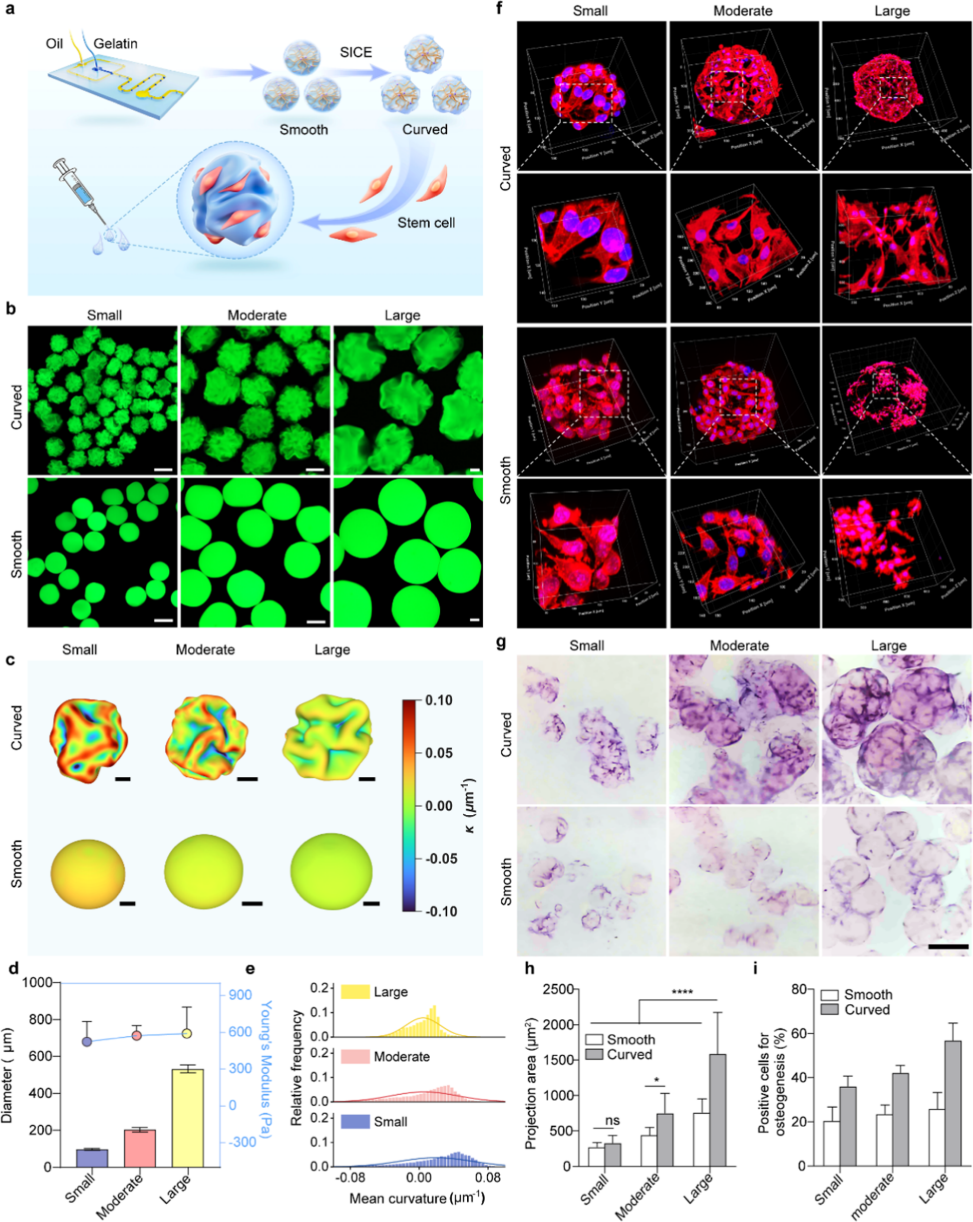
Multiaxial curvature engineering on 3d ultrasoft
